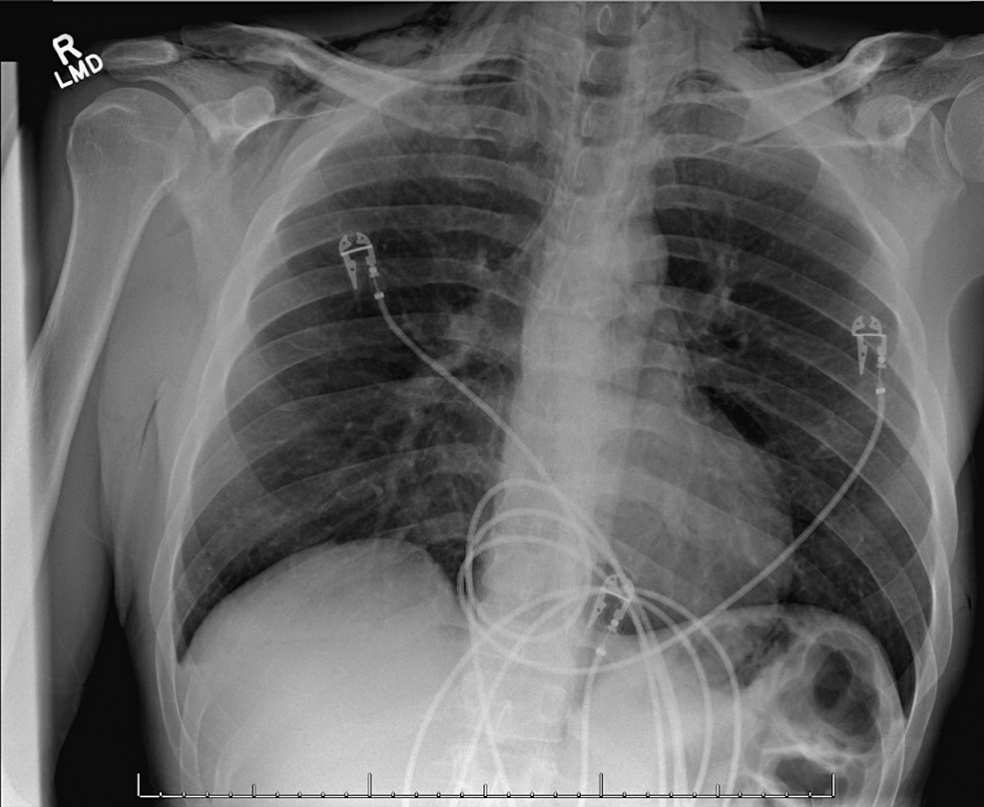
Anterior-posterior chest X-ray demonstrating pneumomediastinum and subcutaneous emphysema
